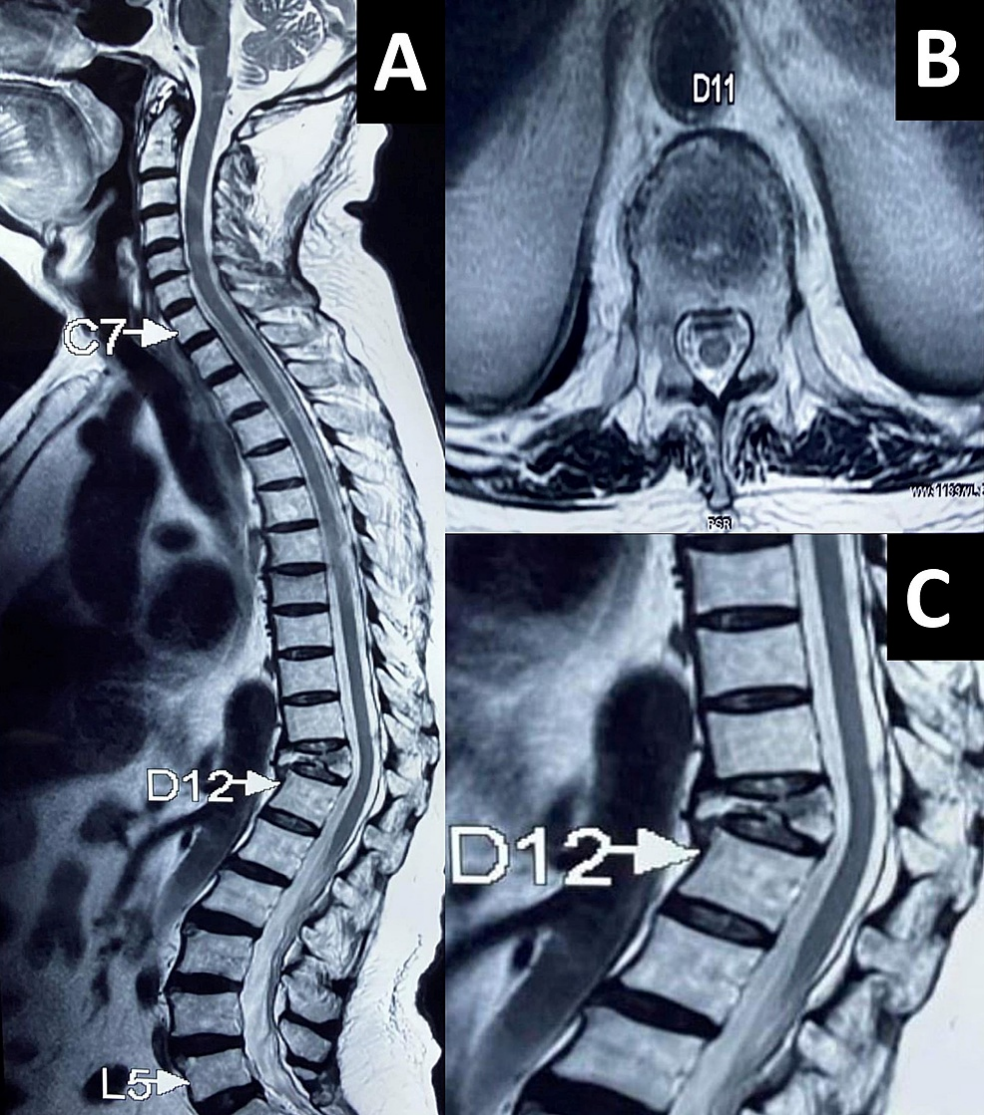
A: T2-weighted longitudinal cross-sectional image of the whole spine with a thoracic D11 vertebral body extensive bony necrotic lesion with complete tuberculous destruction of the body and obliterated disc margins. Also noted was a small grade I compression of the thoracic D7 vertebral body. B: Axial cross-sectional image at thoracic D11 level showing a small posterior epidural retropulsed component just intending the thecal sac. C: Grade III compression fracture of the D11 vertebral body with hypertrophied anterior longitudinal ligaments, but the spinal cord looks spared with no edema or signal intensity changes. Pre- or paravertebral cold abscess or gibbus formation not seen. The discs, pedicles, and lamina also seem to be intact.
